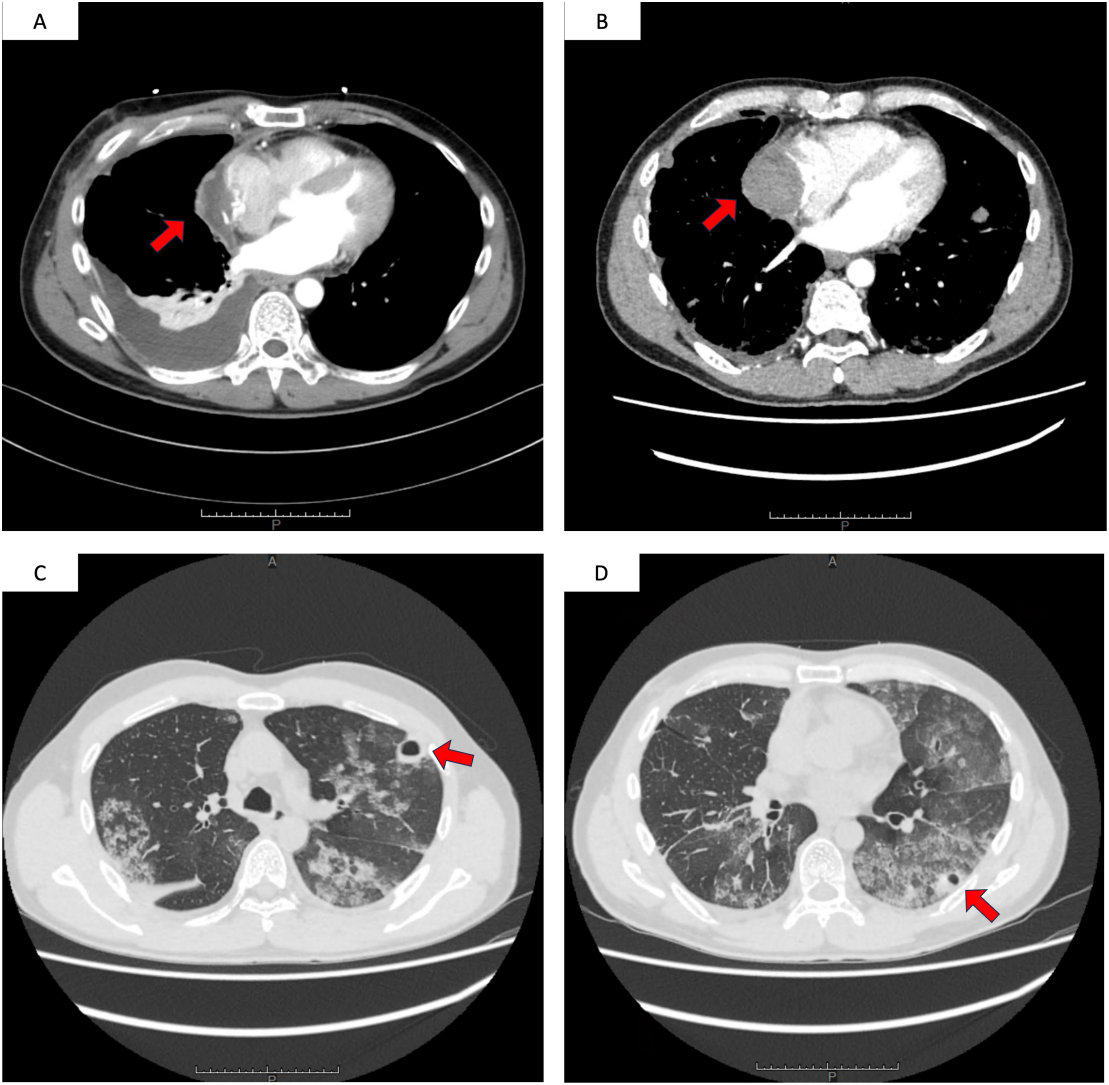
Computed tomography (CT) finding. (A) CT scan at the time of right atrial rupture 2 months earlier. High‐attenuation soft tissue lesion in the right atrium was observed. (B) CT scan at the time of presentation to our hospital. Slight enlargement of high‐attenuation soft tissue lesion in the right atrium was observed. (C and D) CT scan at the time of presentation to our hospital. Diffuse ground‐glass opacities with associated thin‐walled cystic lesions were observed in both lungs.
